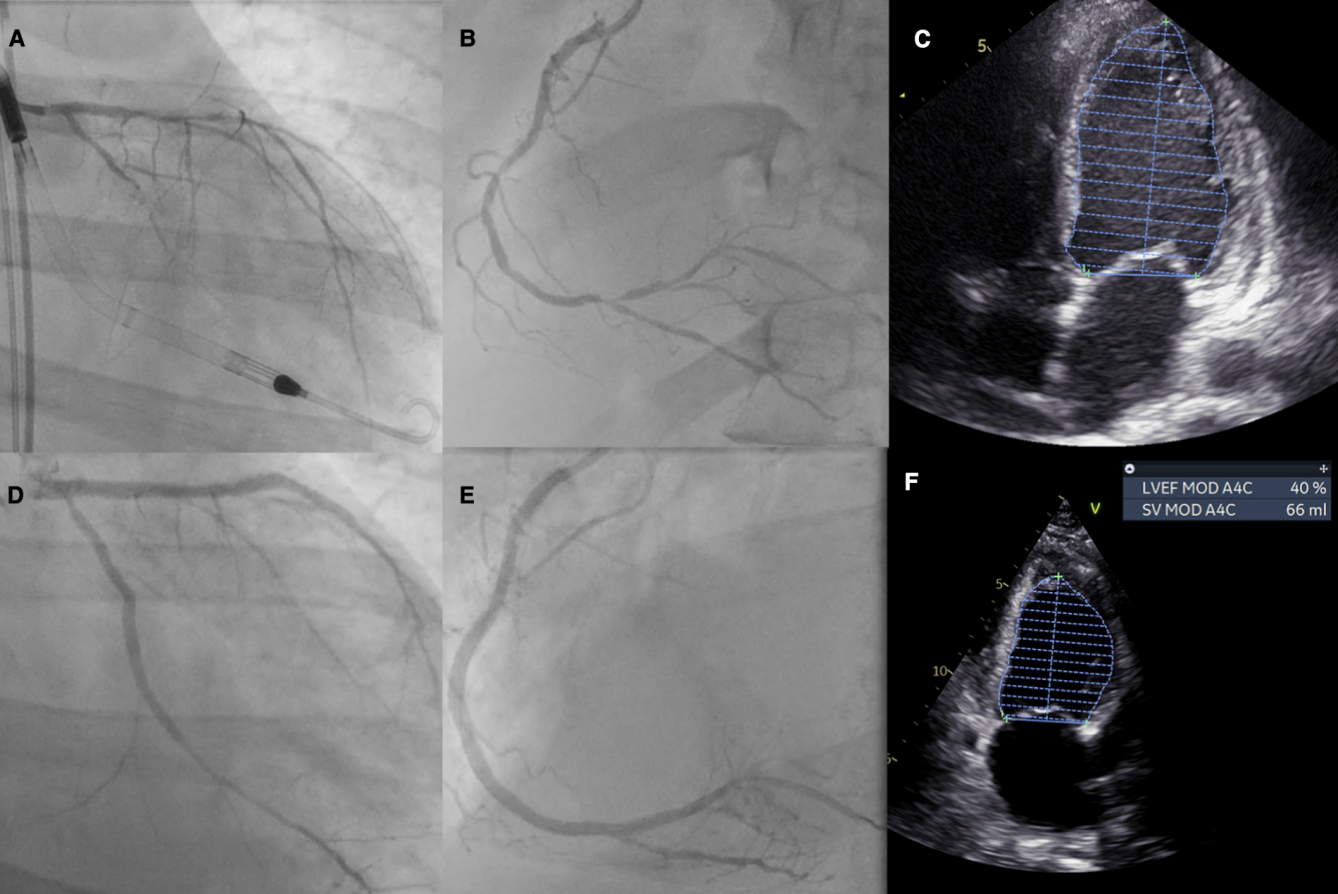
Patient summary: (A) initial left coronary artery anatomy with visible Impella support, (B) initial right coronary artery anatomy, (C) initial echocardiography with an LVEF of 18%, (D) final PCI effect of the left coronary artery, (E) final PCI effect of the right coronary artery, and (F) echocardiography on follow-up with an LVEF of 37%–38%. LVEF, left ventricular ejection fraction.
